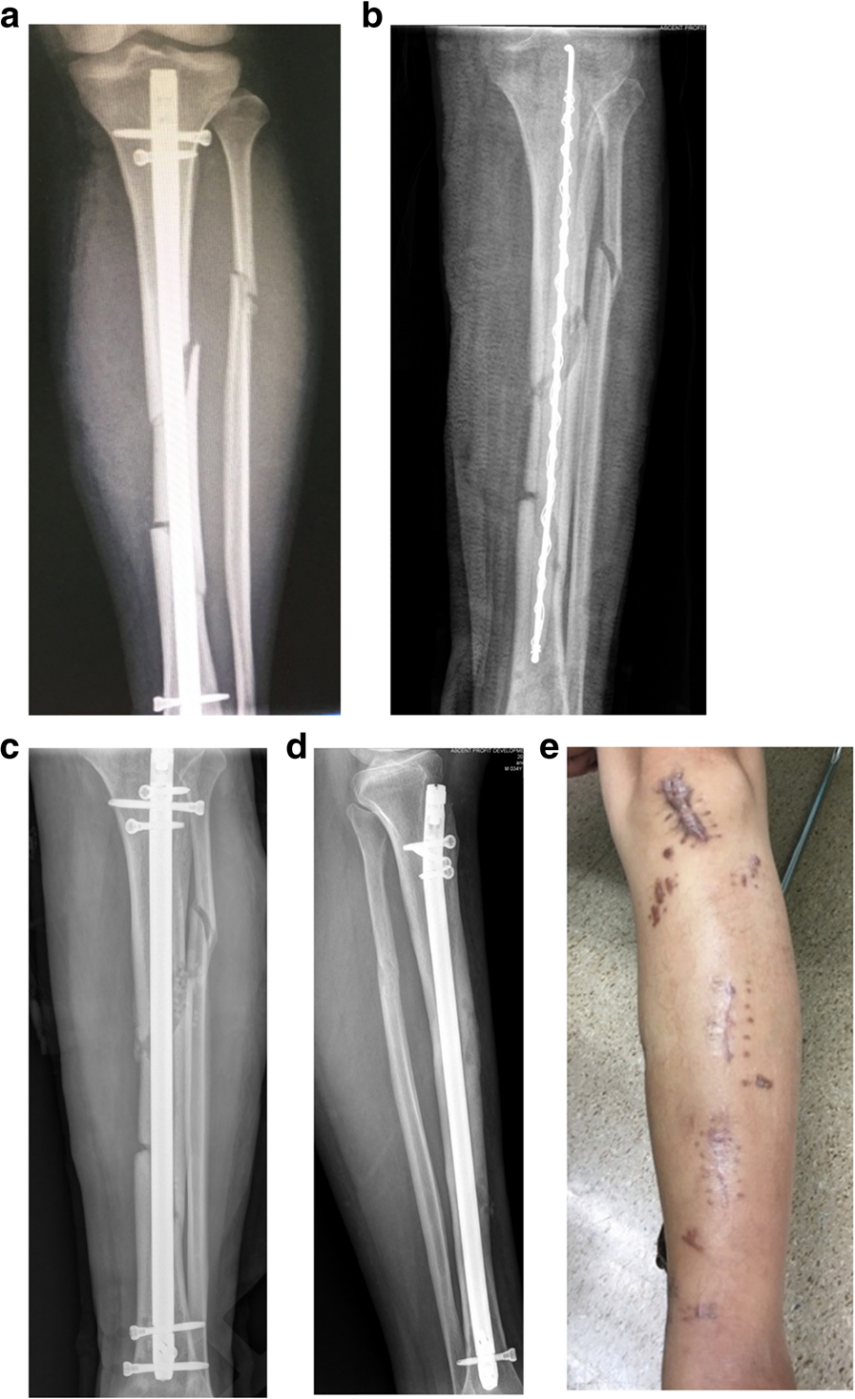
Stable hardware + bone not healed Cierny–Mader 1 type = remove hardware, temporary stabilize + antibiotic cement-coated (ACC) rods, debridement, and reconstruction when clean. a Radiographs of primary internal fixation. b The tibia locking intramedullary nail was removed and replaced by antibiotic cement-coated (ACC) rods. c Reconstruction with locking intramedullary nail with drug delivery system (DDS) when clean. d Radiograph 12 months after treatment. e After 12 months of treatment, the wound was stable
